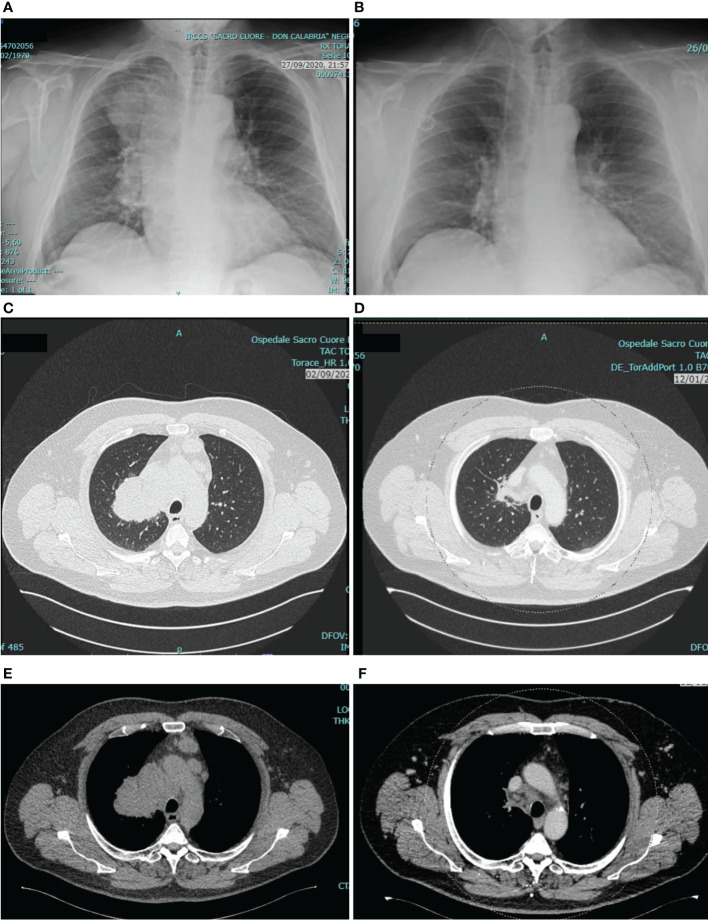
Tumor assessment at baseline and after 4 cycles of pembrolizumab/carboplatin/pemetrexed. The figure shows chest X-ray before (A) and after (B) treatment, and chest CT scan before (C, E) and after (D, F) treatment. As compared with baseline, tumor assessment after 4 cycles revealed a deep response of the primary tumor in the upper right pulmonary lobe, and a reduction of para-tracheal and pre-vascular mediastinal pathologic lymph nodes.
